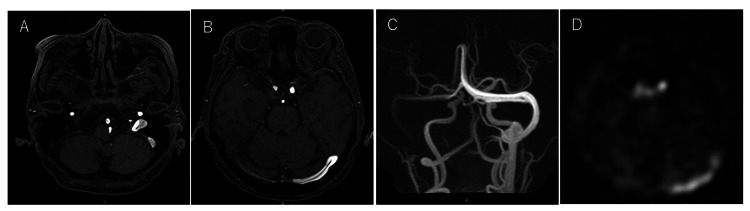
The patient's MRI images Time-of-flight magnetic resonance angiography (3.0 scanner, TR=24ms, TE=2.7ms) showed high signal intensity in the left jugular bulb, sigmoid sinus, transverse sinus, and the proximal side of the sagittal sinus (A, B: source images, C: maximum intensity projection image). The arterial spin labeling image also revealed hyperintensity in the cavernous sinus (D).
